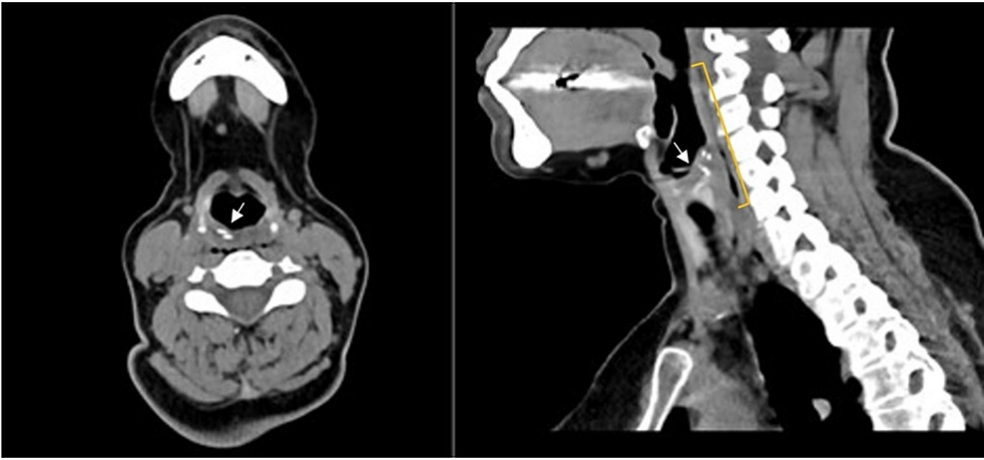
A neck CT scan showing a linear hyperdense foreign body in the hypopharynx (arrows) with a collection of air along the retropharyngeal space (right bracket) representing a concealed perforation
